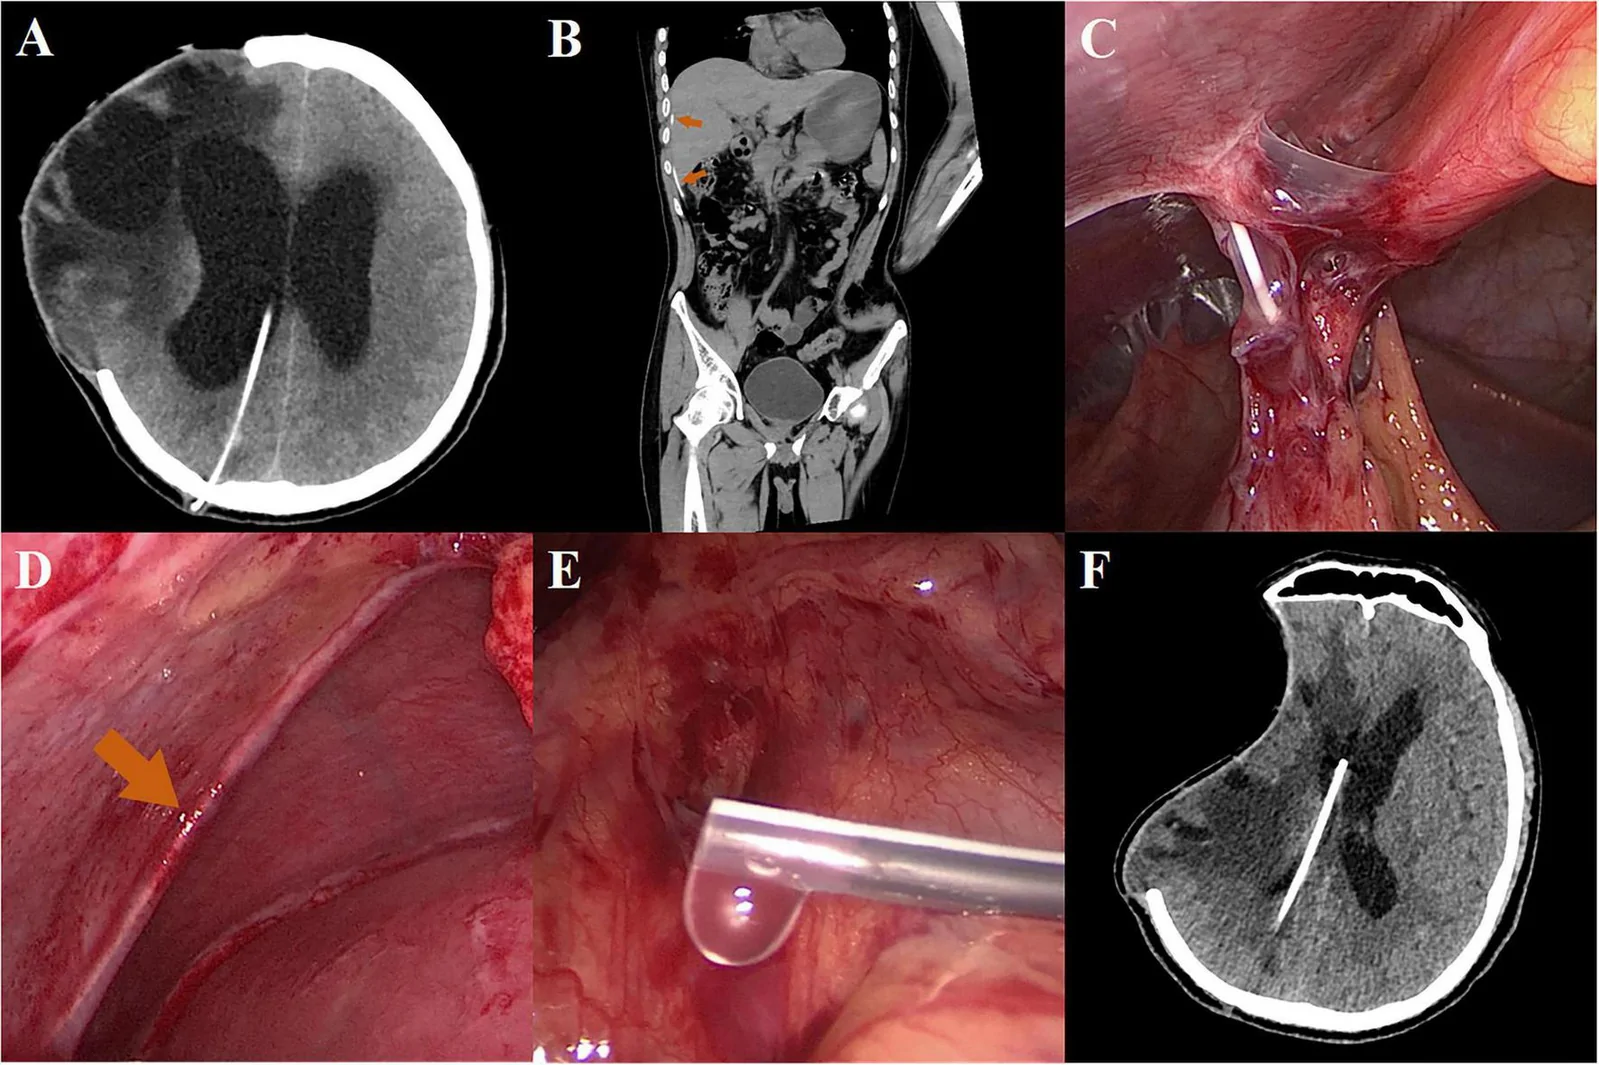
(A) Preoperative axial cranial CT. (B) Preoperative coronal abdominal CT showing the peritoneal catheter coursing along the right hepatic margin toward the inferior surface of the liver. (C) After adhesiolysis on the right side of the round ligament of the liver and exposure of the catheter tract, fluid egress was observed from the fibrous channel. (D) Dissection of the sheath along the hepatic surface. (E) The distal catheter was completely encased within a fibrous sheath and fixed to the parietal peritoneum. (F) Clear CSF outflow was observed after complete skeletonization of the shunt catheter.
